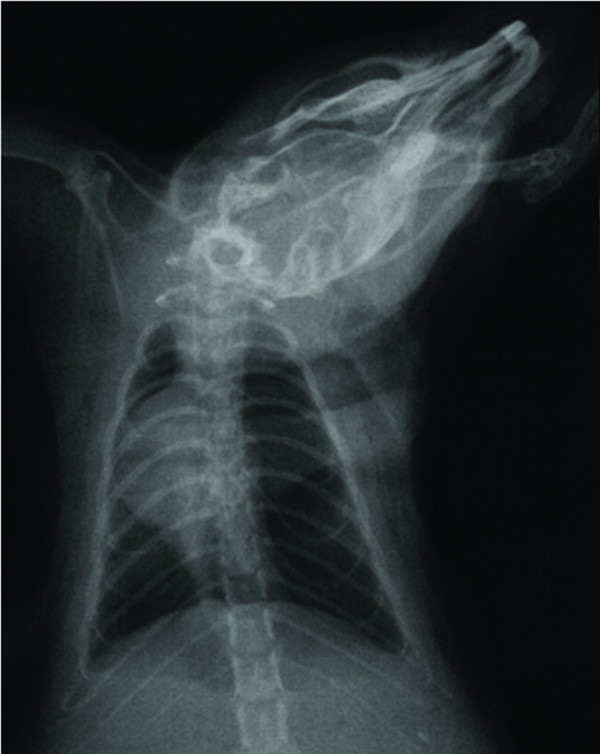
The confirmation of right pneumothorax by chest X-ray.
